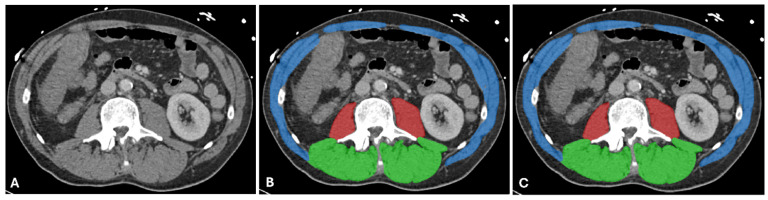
Upper-bound segmentation performance. Representative axial L3 CT slice from the external validation cohort demonstrating optimal model performance in standard cross-sectional anatomy. Panels display the unannotated axial CT (A), manual reference standard (B), and automated ensemble prediction (C). Target classes are color-coded (red = psoas, green = paraspinal, blue = abdominal wall). The overall mean DSC was 0.988. High spatial overlap was achieved across all individual classes: psoas (DSC: 0.992), paraspinal (DSC: 0.991), and abdominal wall (DSC: 0.980). The automated prediction closely aligns with the manual reference standard, without erroneously including adjacent tissues.
